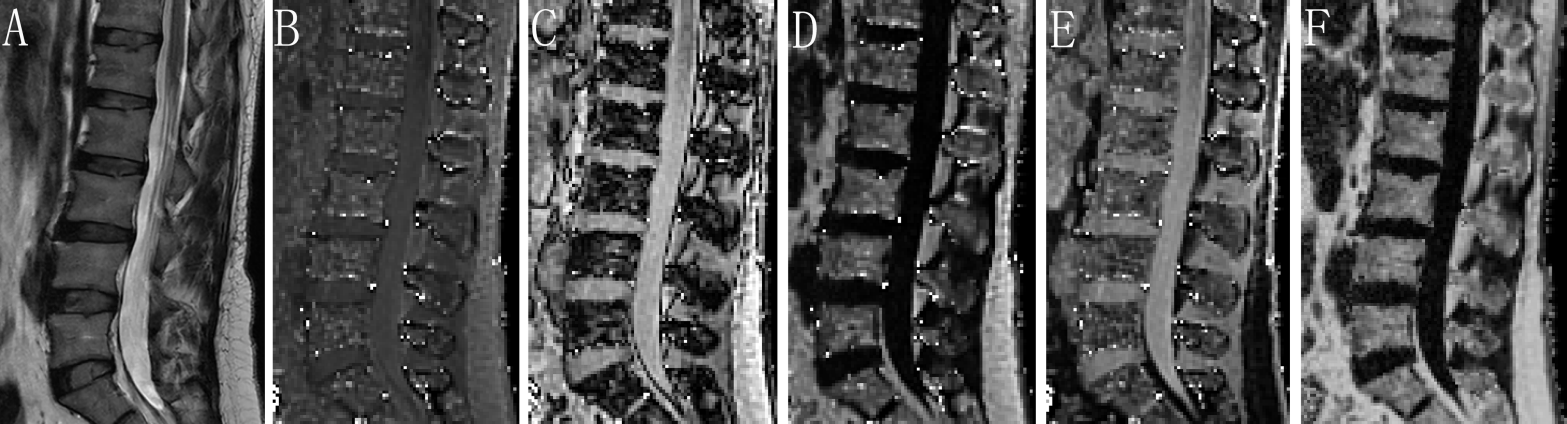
Assessment of PDFF derived from six-echo FACT sequence in image set of a healthy woman. Sagittal T2-weighted (A), in-phase (B), out-of-phase (C), fat-only (D), water-only (E), and the corresponding PDFF map (F) with a mean PDFF of 53% at the L1-L4 levels. FACT, Fat Analysis & Calculation Technique imaging; PDFF, proton density fat fraction.
